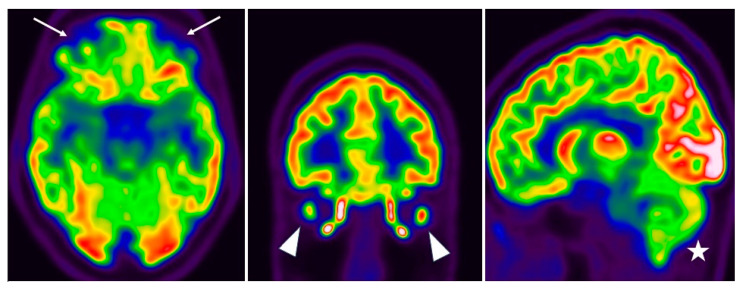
The 18F-FDG PET on axial, coronal and sagittal planes showed bilateral hypometabolism in the inferior frontal lobe (olfactory gyrus) (arrow), in the temporal lobe (limbic/paralimbic regions) (arrowhead) and in the cerebellum (star).
